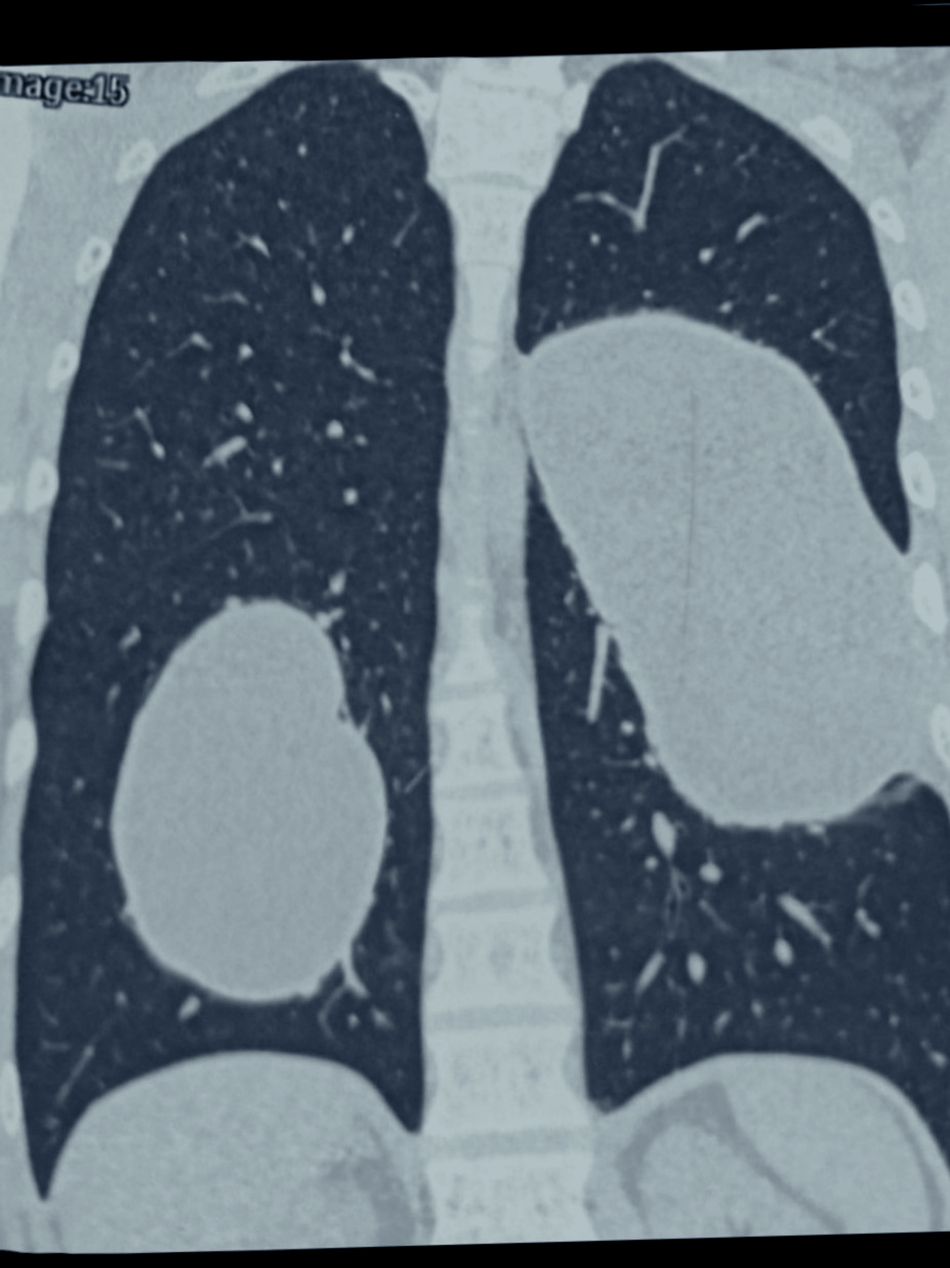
CECT chest showing hypodense, non-enhancing cystic lesions in bilateral lungs suggestive of hydatid cysts in the lungs CECT: contrast-enhanced computed tomography
